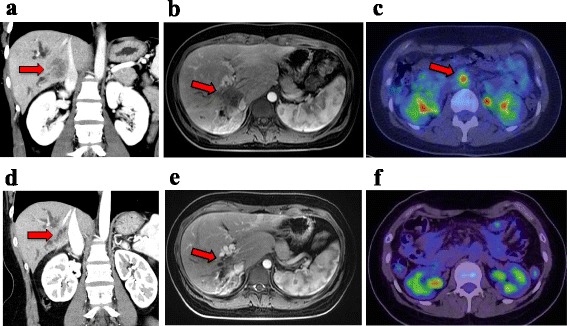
Radiological examinations before (a, b, and c) and after (d, e, and f) downsizing chemotherapy. a, b Computed tomography (CT) and magnetic resonance imaging (MRI) images of the liver showed focal dilation of the right intrahepatic bile duct and a 42-mm tumor in hepatic segments 1 to 8; the tumor was attached to the inferior vena cava (IVC) (a axial view of CT, b coronal view of MRI). c Positron emission tomography (PET) showed fluorodeoxyglucose (FDG) uptake in the para-aortic lymph nodes. d, e The size of tumor decreased to 18 mm, and the tumor was no longer attached to the IVC (d axial view of CT, e coronal view of MRI). f No FDG uptake was seen in the para-aortic lymph nodes
